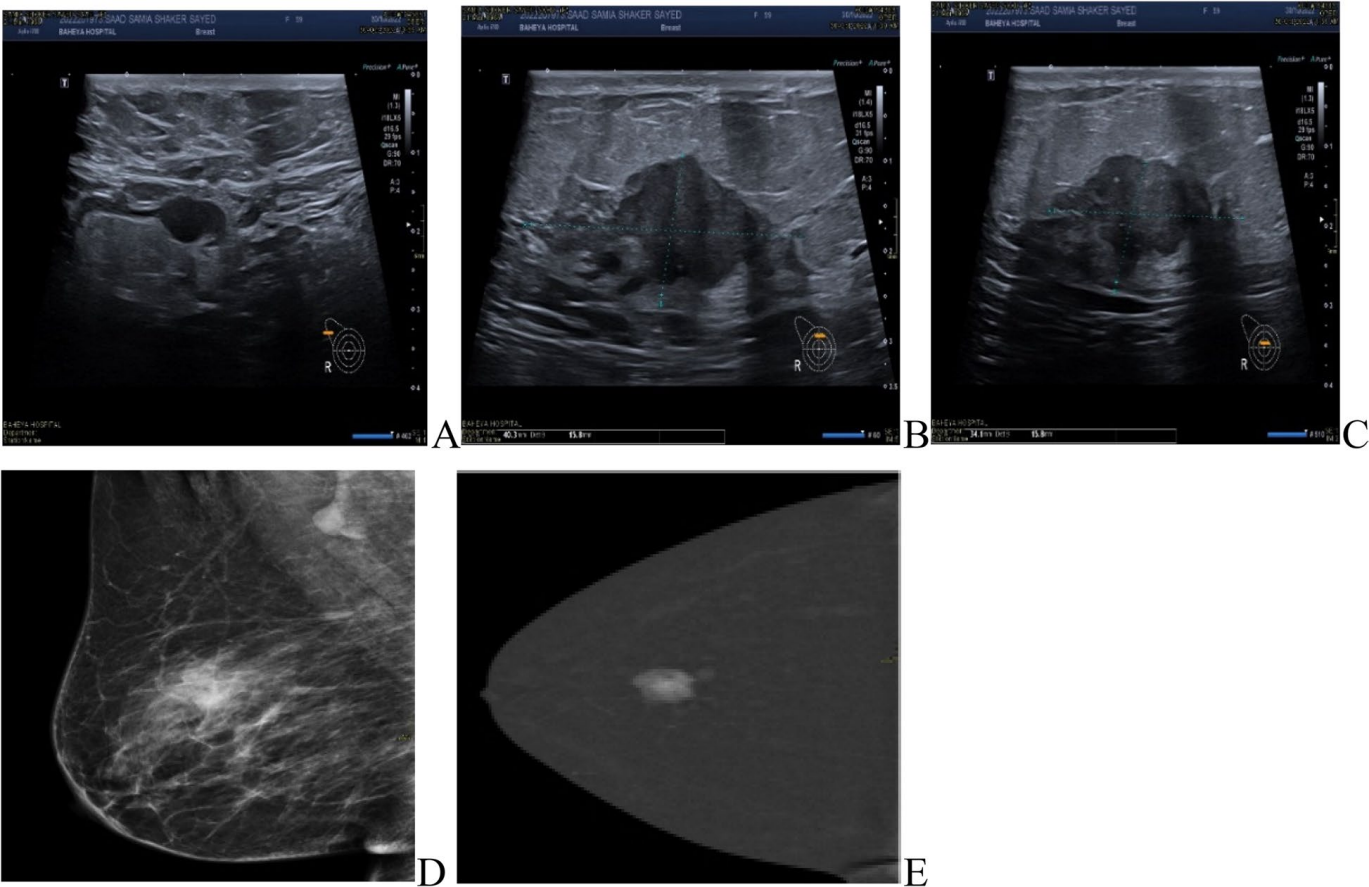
Further imaging modalities. A, B, C 60-years-old female patient had right breast irregular hypoechoic solid mass by ultrasound (BIRADS 5). D, E CESM showed a right breast irregular heterogeneously enhancing solid mass
